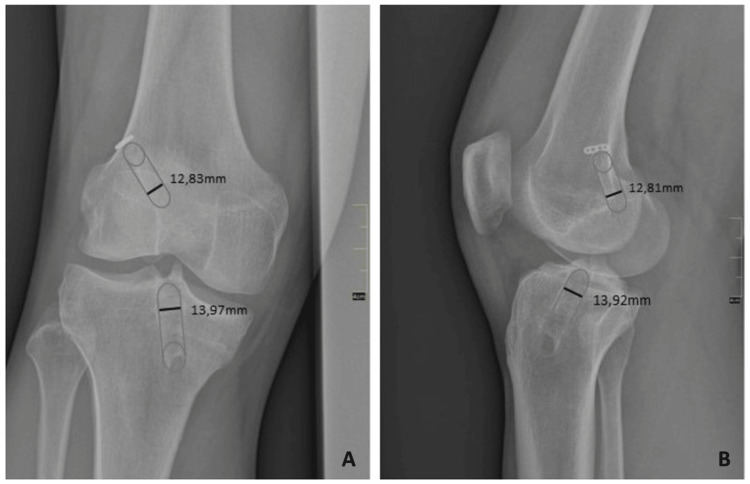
Anteroposterior (A) and lateral (B) knee radiographs showing the method of measuring the femoral and tibial tunnel diameter.
